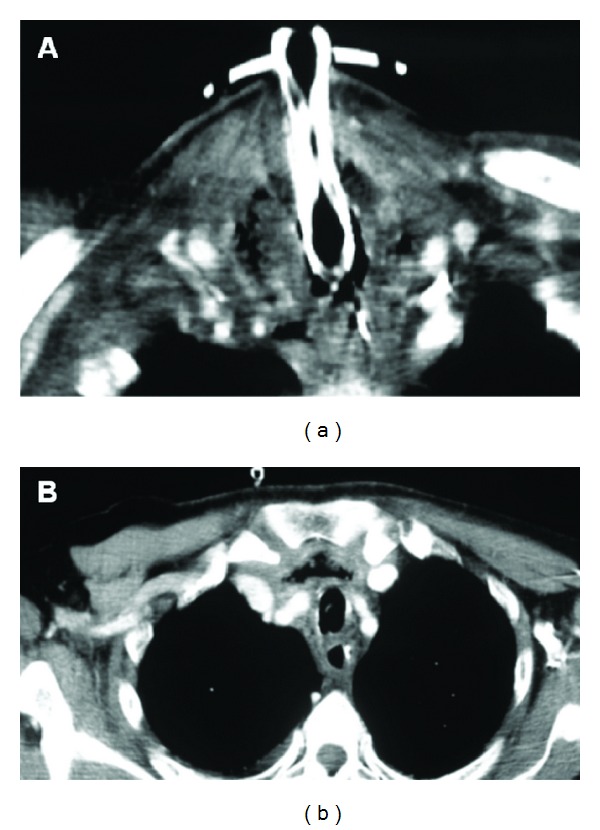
Patient number 2—CT images. (a) Pools of liquid/gaseous material in the neck planes, around the trachea, can be seen. (b) Mild expansion of the upper mediastinum can be observed. A large pool of liquid and gaseous material is easily visible in the retrosternal area.
